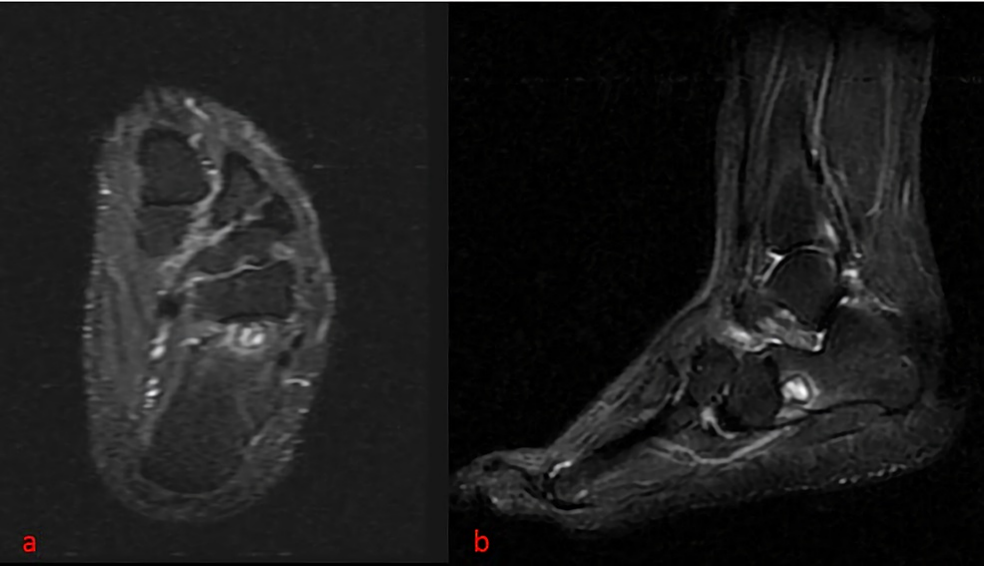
High signal intensity of the lesion in the MRI T2 axial series (a) and (b) sagittal series.
